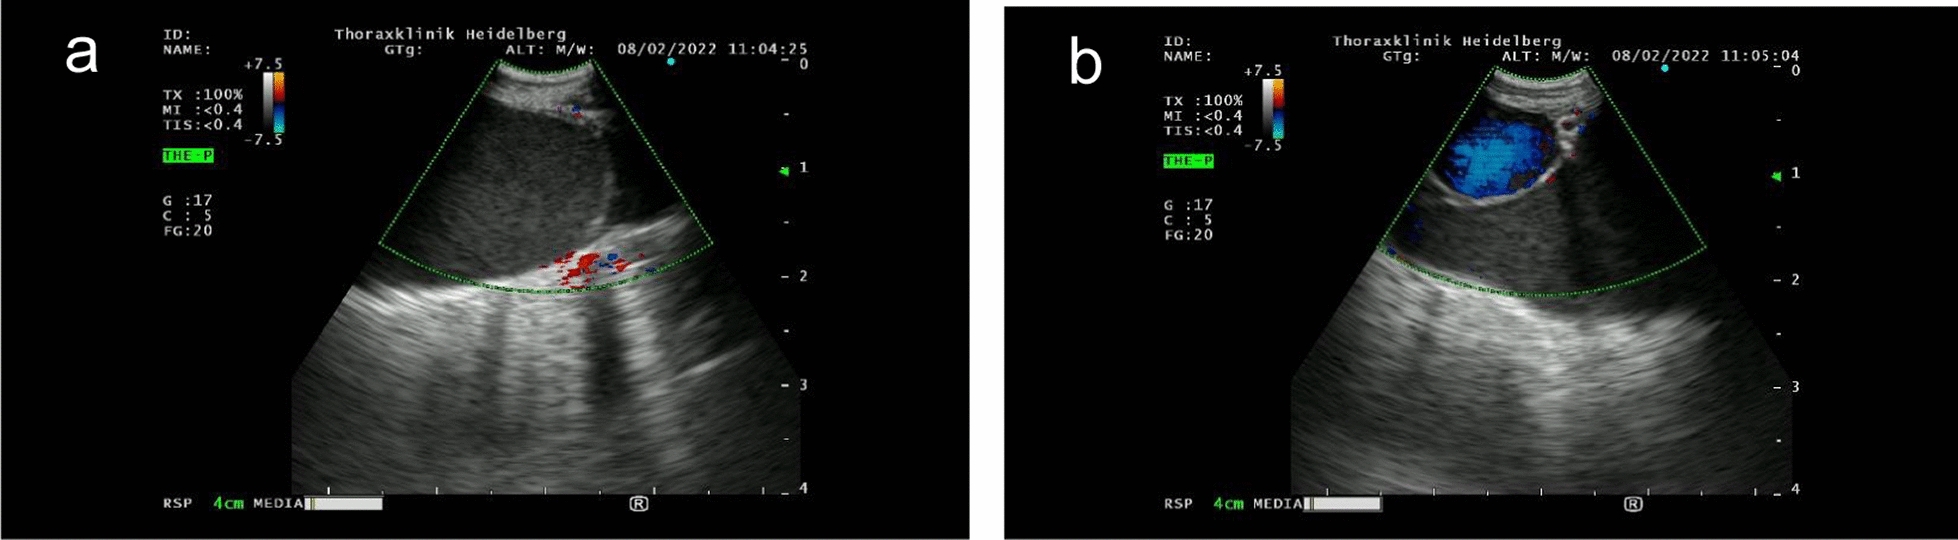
1a and 1b: Endobronchial ultrasound of mediastinal mass showing dilated low-flow non-blood vessels
